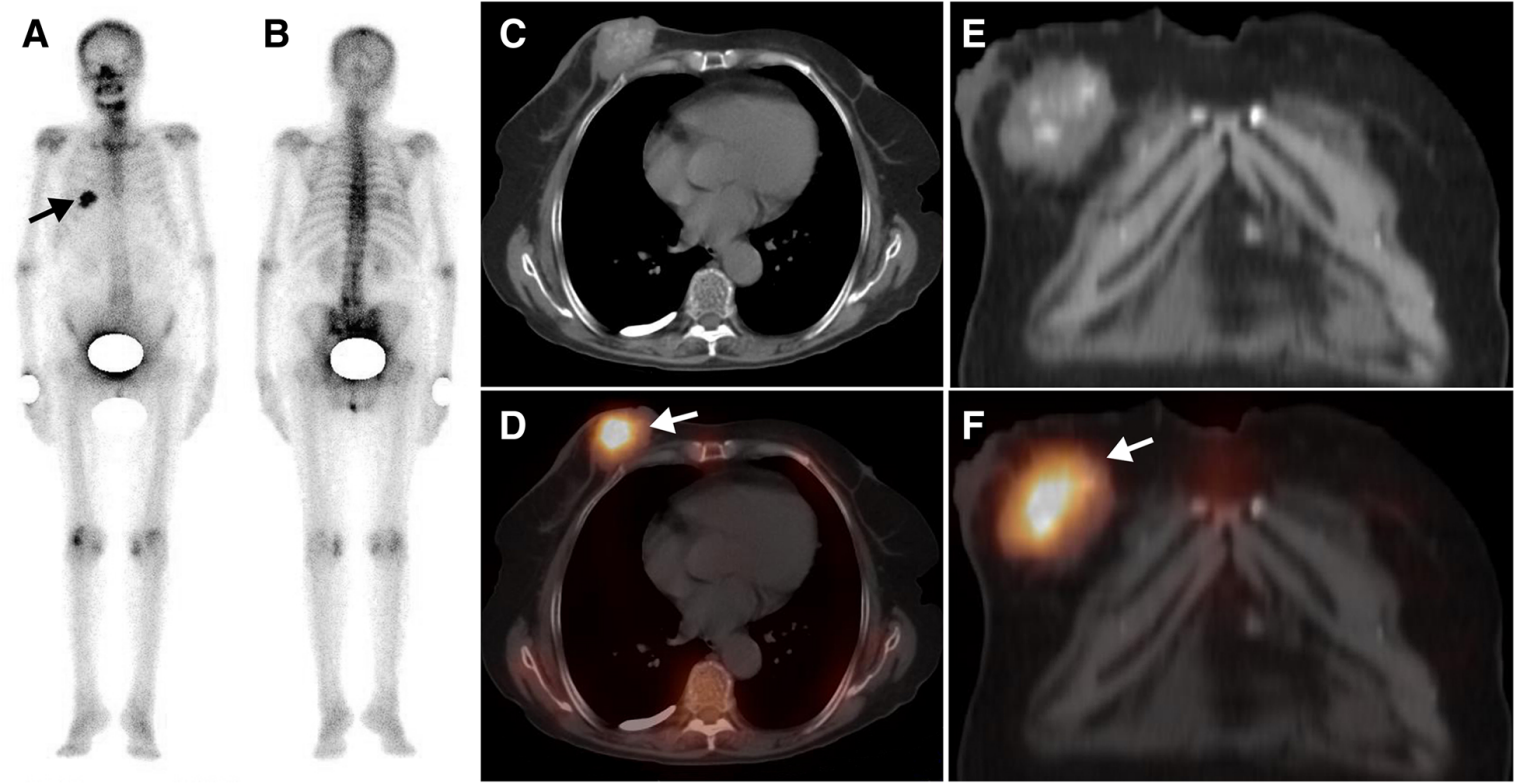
A 76-year-old woman with breast cancer was referred for metastatic work-up before surgery. The whole-body scan (a, anterior; b, posterior) revealed a focal area of high ^99m^Tc-MDP activity in the right 5-6th posterior rib region (black arrow). Axial CT (c), and coronal CT (e) and SPECT/ CT images (d, f) showed that elevated ^99m^Tc-MDP activity was located in a breast tumour with calcification (white arrow)
